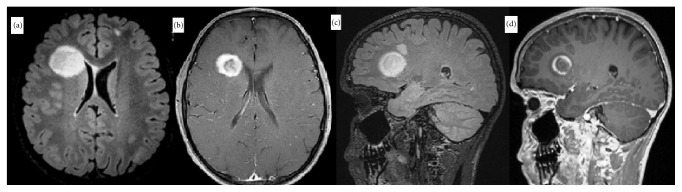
One month later. ((a),(c)) Improvement of the tumefactive lesion is visible in the left frontal lobe and a new tumefactive lesion has appeared in the right frontal white matter. ((b),(d)) T1-weighted postcontrast images show ring enhancement.
